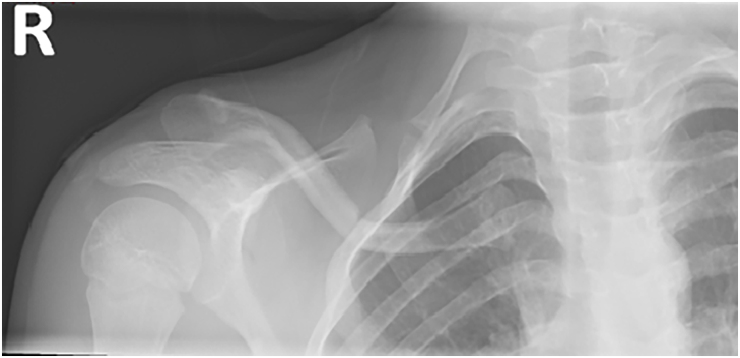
Right clavicule antero-posterior x-ray taken on initial admission to the emergency department
